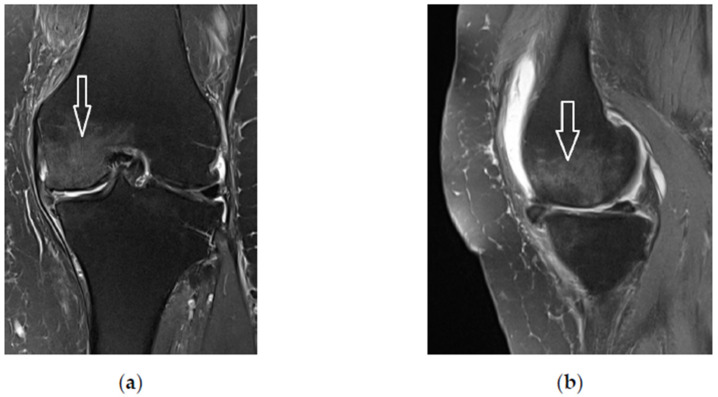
T2-weightened magnetic resonance imaging (MRI) of the left knee. Note bone marrow edema of the medial femoral condyle (arrows). (a) Coronal plane; (b) sagittal plane.
